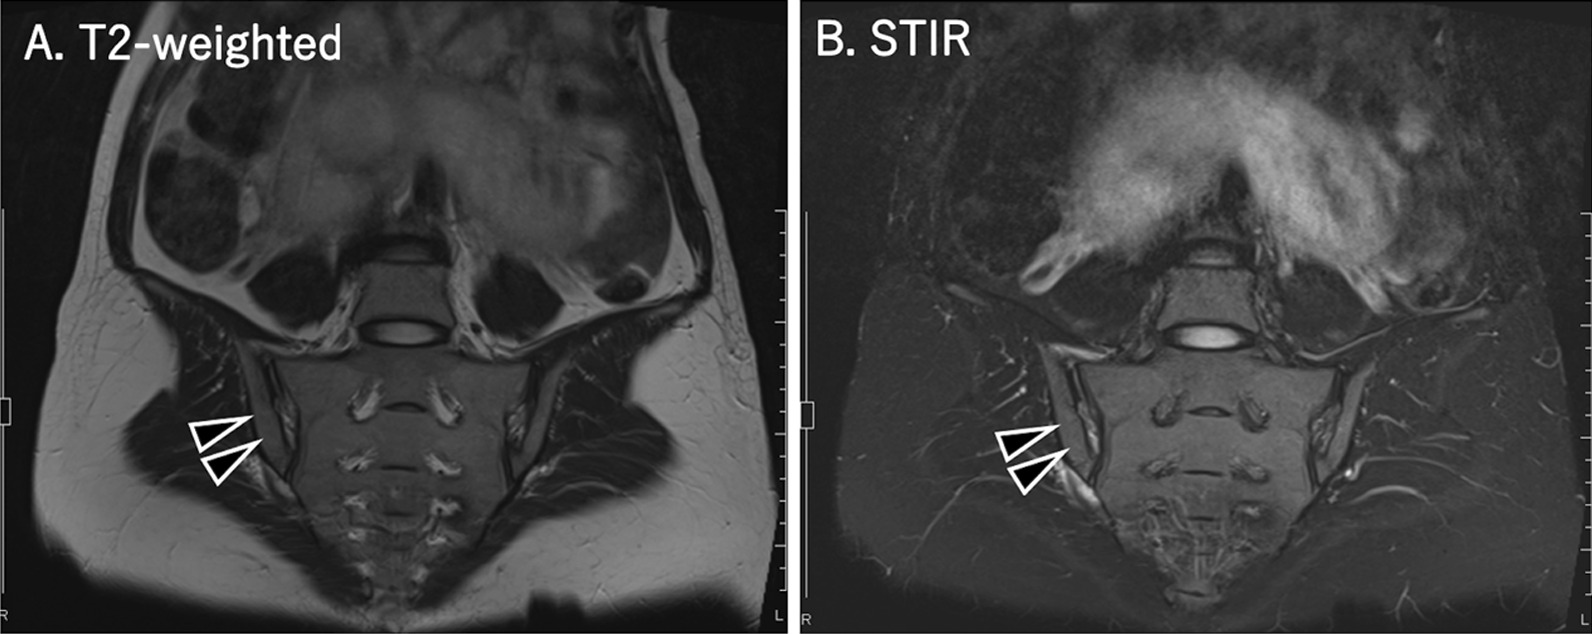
Magnetic resonance imaging scans of the sacroiliac joints of the pregnant patient with right-sided infectious sacroiliitis at the time of hospitalization. A T2-weighted (T2W), B Short-tau inversion recovery (STIR). Arrow head shows high-intensity signal area in the right sacroiliac joint
